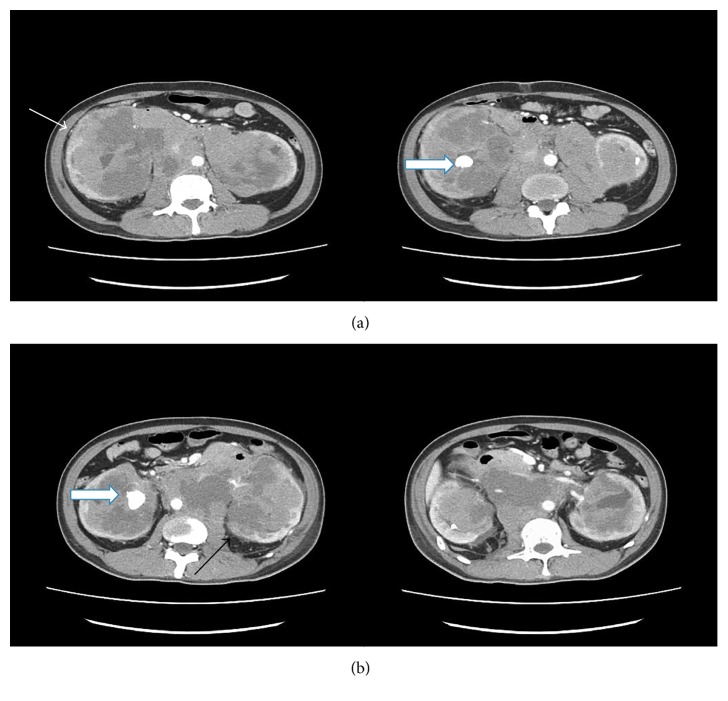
Four-phase renal computed tomography (CT) showed both kidneys were enlarged with the right kidney measuring 18 cm (white arrow) and the left kidney 14 cm (black arrow). There was a concomitant large renal calculus bilaterally, with the largest on the right measuring 1.5 cm × 2.0 cm × 2.2 cm (thick white arrow) and the left measuring 2.2 cm × 1.7 cm × 1.0 cm in the renal pelvis. Smaller renal calculi are seen in the rest of the pelvicalyceal systems.
